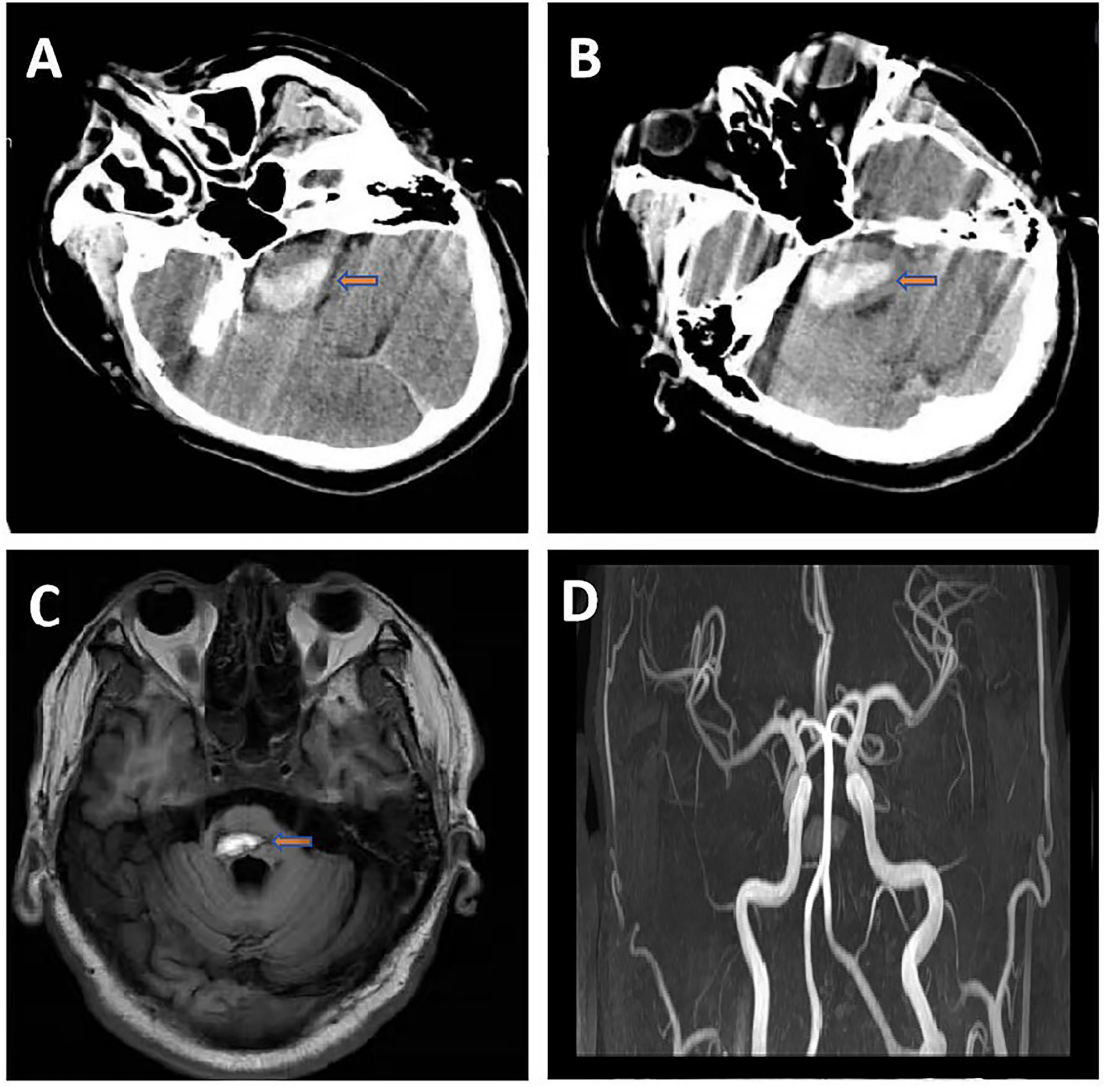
(A,B) Cranial computed tomography showed high-density shadow in the brainstem (volume 10.3 ml, CT value 53.6 Hu) with surrounding edema (volume 5.2 ml, CT value 33.9 Hu) consistent with acute brainstem hemorrhage. (C) Cranial magnetic resonance imaging showed hypointense ring-like signal around the lesion on T2-weighted images, indicating chronic phase changes of brainstem hemorrhage in the right paramedian region. (D) Cranial magnetic resonance angiography showed the bilateral internal carotid arteries, middle cerebral arteries, anterior cerebral arteries, basilar artery, and major branches of the posterior cerebral arteries have a natural course, with no stenosis or deformation.
